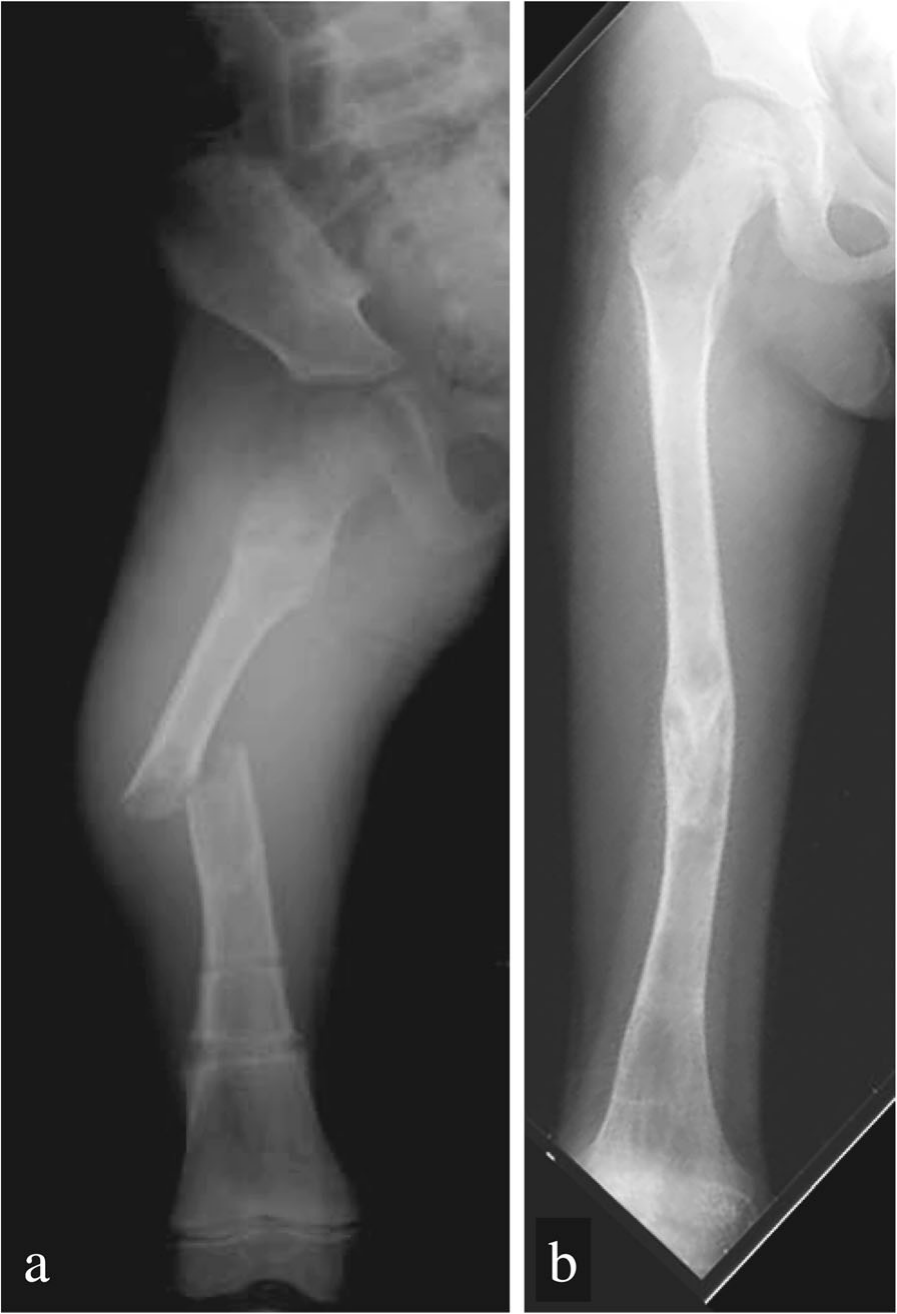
Right femur frontal radiographs. a Second fracture, at 1 week after removing the external fixation. b At the time, when bone fracture had healed using conservative therapy
